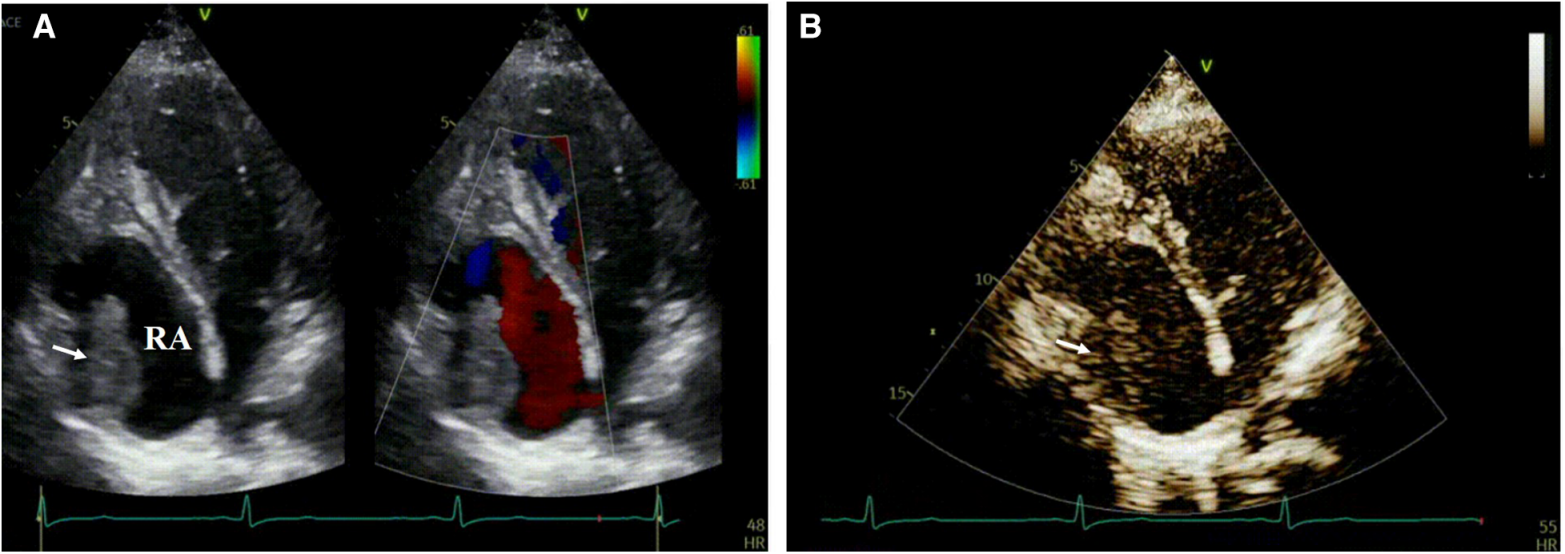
Depicts the right atrium in transthoracic echocardiography with the annotation that RA represents the right atrium. In (A) an echogenic mass is visualized within the right atrium (indicated by arrows), while color Doppler flow imaging reveals no blood flow signal within the mass. (B) Demonstrates that contrast-enhanced cardiac imaging does not exhibit significant enhancement of the aforementioned mass.
